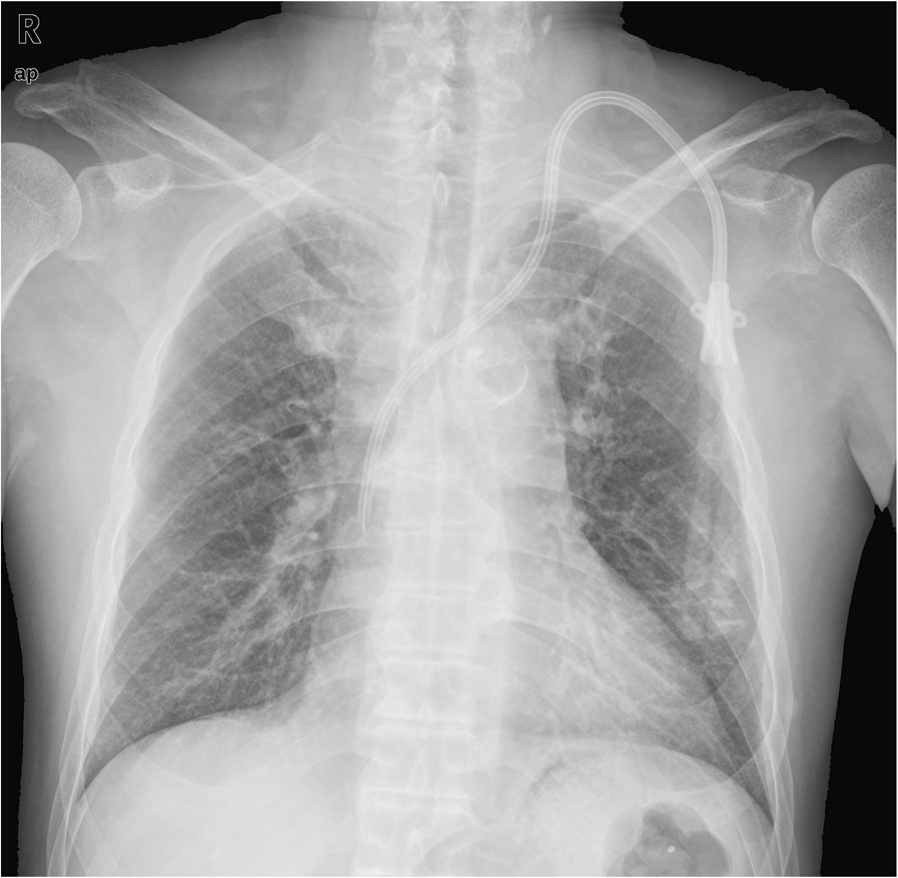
The chest radiograph of the cuffed, tunneled hemodialysis catheter used now
